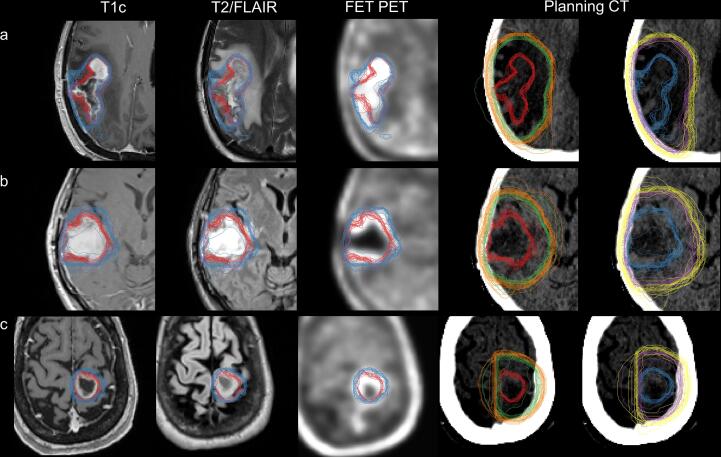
An overview of all Radiation Oncology target volume (TV) delineations on axial imaging. Each row represents the benchmarking cases FET1CASE1 (a), FET1CASE2 (b), and FET1CASE3 (c). Standard GTV_MR_ (red), CTV_MR_ (green), PTV_MR_ (orange), and hybrid GTV_MR+FET_ (blue), CTV_MR+FET_ (purple), PTV_MR+FET_ (yellow) are shown. For comparison, GTV_MR_ and GTV_MR+FET_ are shown together with T1c, T2/FLAIR, and FET PET with all images co-registered to each cases respective planning CT where all TV_MR_ and TV_MR+FET_ are displayed separately. (For interpretation of the references to colour in this figure legend, the reader is referred to the web version of this article.)
